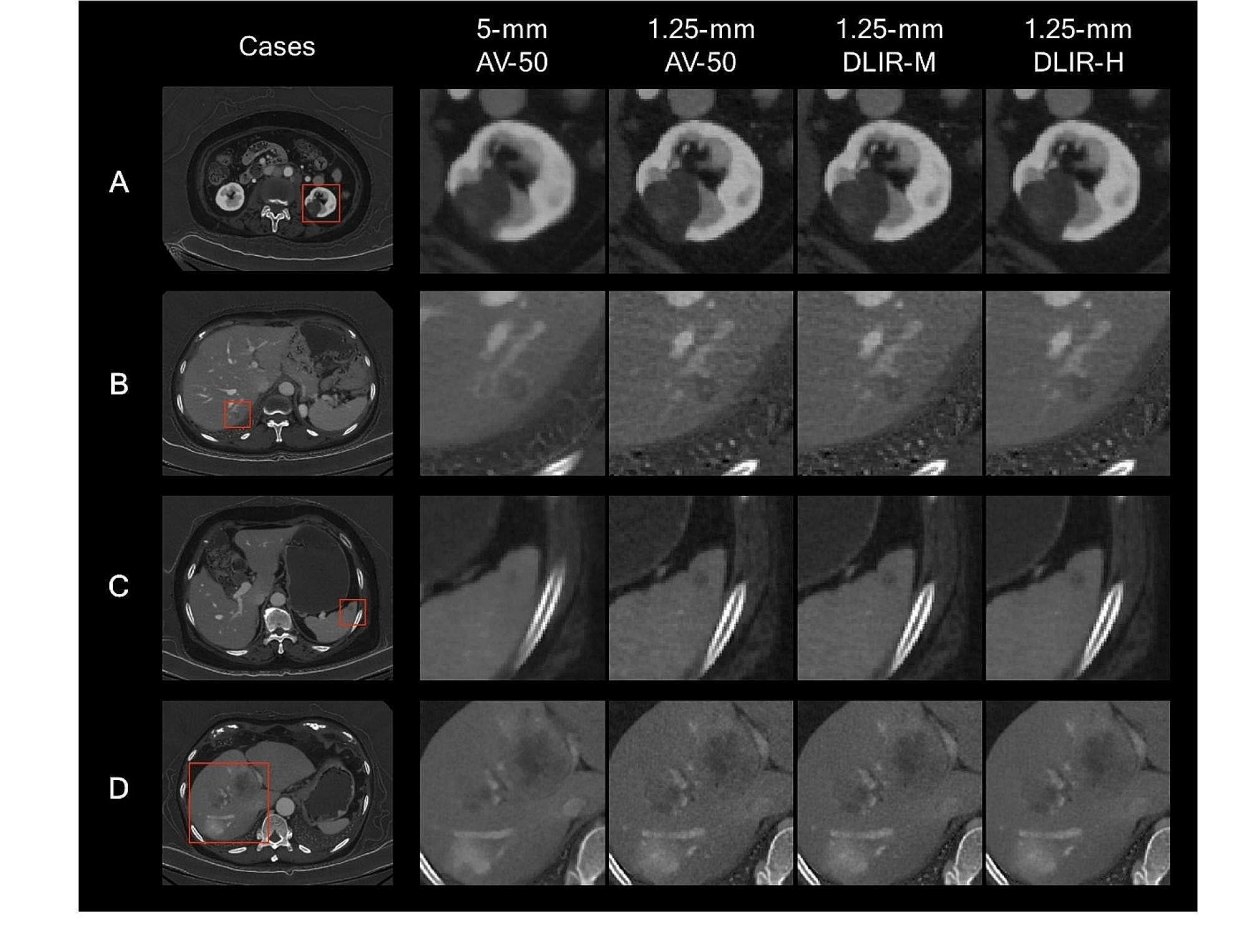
Four examples of abdominal CT studies with measurements. These portal-venous phase abdominal CT studies were reconstructed into iodine maps using 5-mm AV-50, 1.25-mm AV-50, 1.25-mm DLIR-M, and 1.25-mm DLIR-H algorithms, respectively; and present with the same windowing (width of 15.0 mgI/mL, level of 5.0 mgI/mL). (A) A patient was scanned for a suspected left renal lesion. With 1.25-mm images, the internal enhancement was better detected. The difference of iodine concentration between the low-density compartment and subtle enhancement was more obvious with 1.25-mm AV-50 (1.14 mgI/mL) and 1.25-mm DLIR-H (1.04 mgI/mL) images, than 5-mm AV-50 (0.68 mgI/mL) image, which suffered from the partial volume effects. (B) A patient was scanned for cancer staging. The hepatic lesion with subtle circular and center enhancement in with 5-mm AV-50 image was suspected to be a metastasis lesion. The 1.25-mm images better visualized the boundary of lesion, and the subtle circular enhancement was excluded. The detailed center enhancement in the lesion can be better depicted in 1.25-mm images. The ERS of border of the enhancement was higher in 1.25-mm AV-50 [0.437 (mgI/mL)/mm] image than 5-mm AV-50 [0.262 (mgI/mL)/mm] image, and was even higher in 1.25 DLIR-H [0.655 (mgI/mL)/mm] image. The follow-up scans indicated that the lesion is benign. (C) A patient was scanned for a lesion in spleen. The difference of iodine concentration between the low-density lesion and spleen parenchyma was more obvious with 1.25-mm AV-50 (1.14 mgI/mL) and 1.25-mm DLIR-H (1.04 mgI/mL) images, than 5-mm AV-50 (0.60 mgI/mL) image. The ERS of the lesion boundary was higher in 1.25-mm AV-50 [0.334 (mgI/mL)/mm] and 1.25 DLIR-H [0.359 (mgI/mL)/mm] images than 5-mm AV-50 [0.164 (mgI/mL)/mm] image. The 1.25-mm DLIR-H image with lower noise improved the diagnostic confidence of a cyst. (D) A patient with gastric cancer was scanned for hepatic metastases. With the 1.25-mm images, the left boundary of the lesion was clearer than the 5-mm AV-50 images. The ERS of the subtle left boundary was higher in in 1.25-mm DLIR-H [0.586 (mgI/mL)/mm] image than 1.25 AV-50 [0.373 (mgI/mL)/mm] image, and lowest in 5-mm AV-50 [0.1.66 (mgI/mL)/mm] image. It was hard to measure the size of the lesion in 5-mm image, while it can be easier to be measured in 1.25-mm images, to guide later treatment selections
